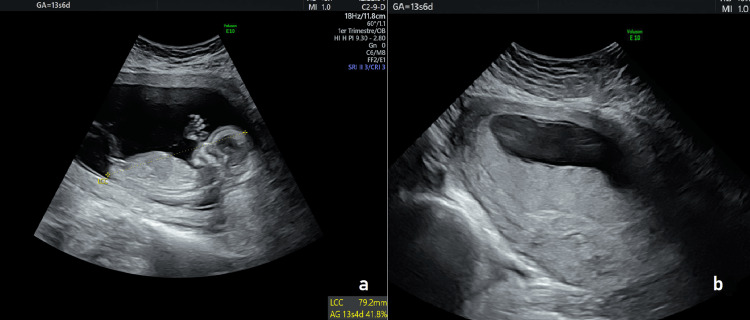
First-trimester obstetric ultrasounds. (a) Longitudinal crown–rump view showing a single intrauterine gestation. (b) Placenta with normal shape and location, without evidence of abnormalities.
